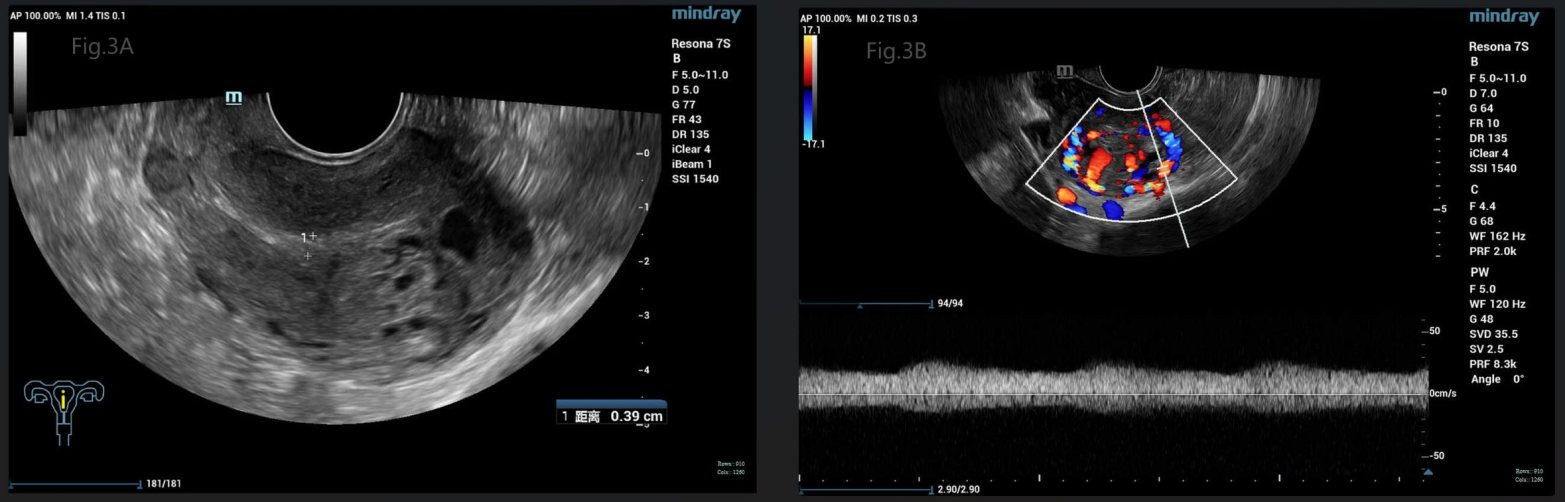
(A) Gray-scale pelvic sonography showed a large honeycomb like mass within the uterine fundus, close to the serosa. (B) Pulsed-wave Doppler detected a high-speed, low-resistance arteriovenous fistula spectrum.
